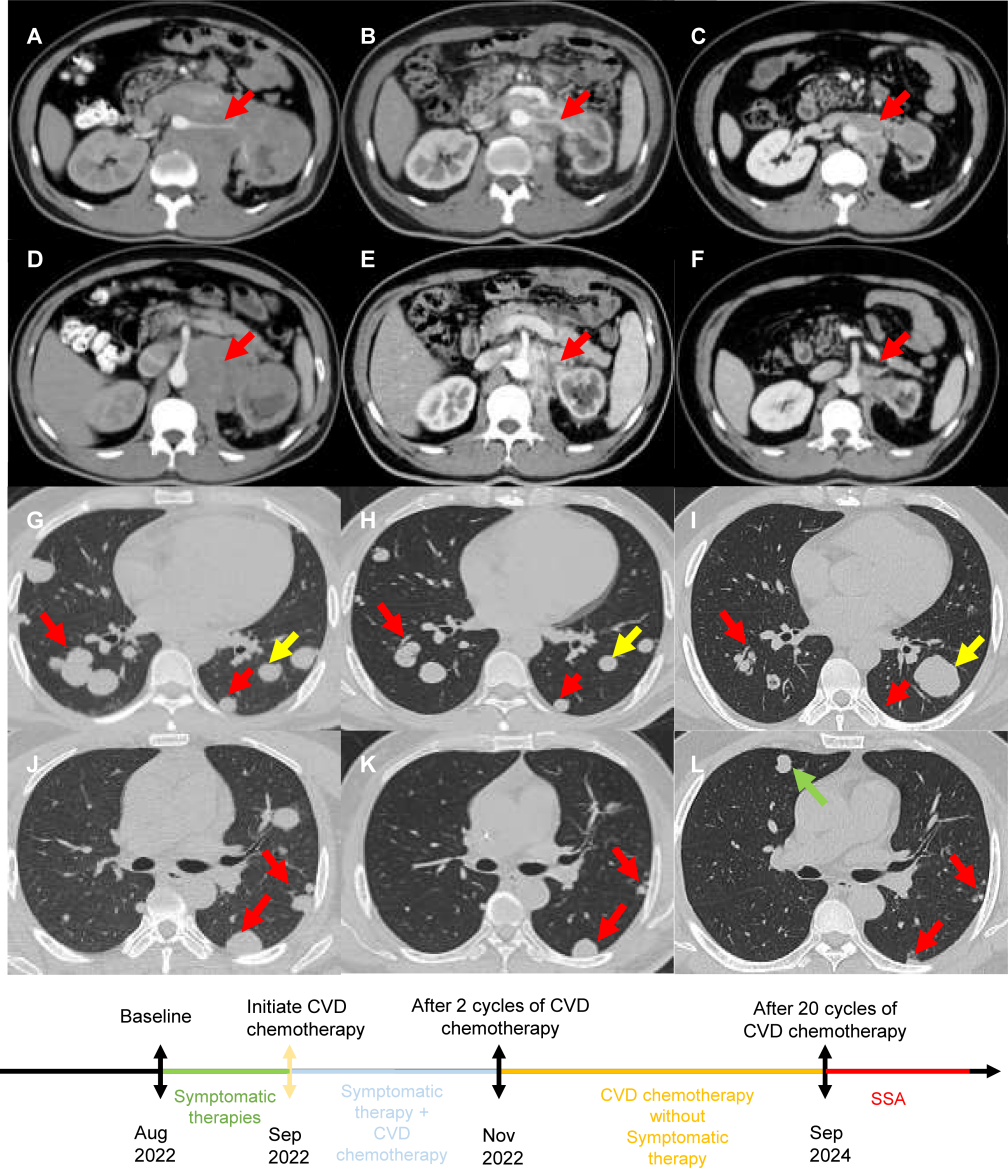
(A, D, G, J) Prior to CVD chemotherapy, CT revealed retroperitoneal lesions around the pancreas, liver, kidney and multiple diffuse lesions in both lungs. (B, E, H, K) After 2 cycles of CVD chemotherapy, CT revealed that retroperitoneal lesions around the pancreas, liver, kidney and multiple diffuse lesions in both lungs reduced in size. (C, F, I, L) After 20 cycles of CVD chemotherapy, CT revealed retroperitoneal lesions further reduced in size. Although a small amount of lung lesions enlarged and new lesions appeared, the majority of lung lesions reduced in number and size. [Red arrows indicate shrinking lesions, yellow arrows indicate enlarged lesions, and green arrows indicate new lesions.].
